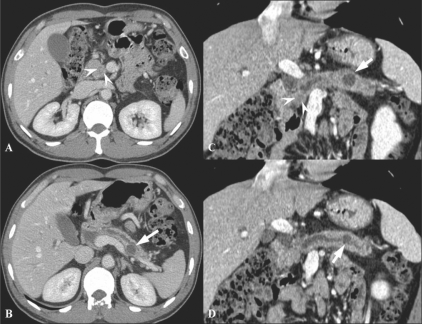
A 44-year-old man with pancreatic head cancer and a pseudocyst in tail. Axial CT scan (A) shows an ill-defined low attenuated mass (arrowheads) in the uncinate process of the pancreas. The main pancreatic duct is diffusely dilated (B) with another cystic lesion in the pancreatic tail (arrow in B). Curved MPR images (C,D) show better appreciation of the tumor (arrowheads in C) and the tail cyst (arrows in C and D), including its communication with the main pancreatic duct (arrow in D)
